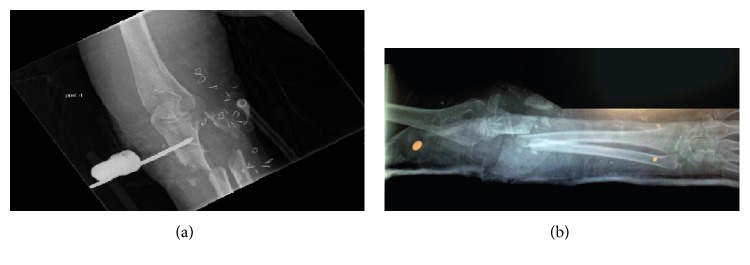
(a) AP X-ray after application of external fixator device demonstrating bony injury on initial injury after debridement. (b) AP X-ray showing the bony injury involving the ulna and radius, with a large amount of radial bone loss.
